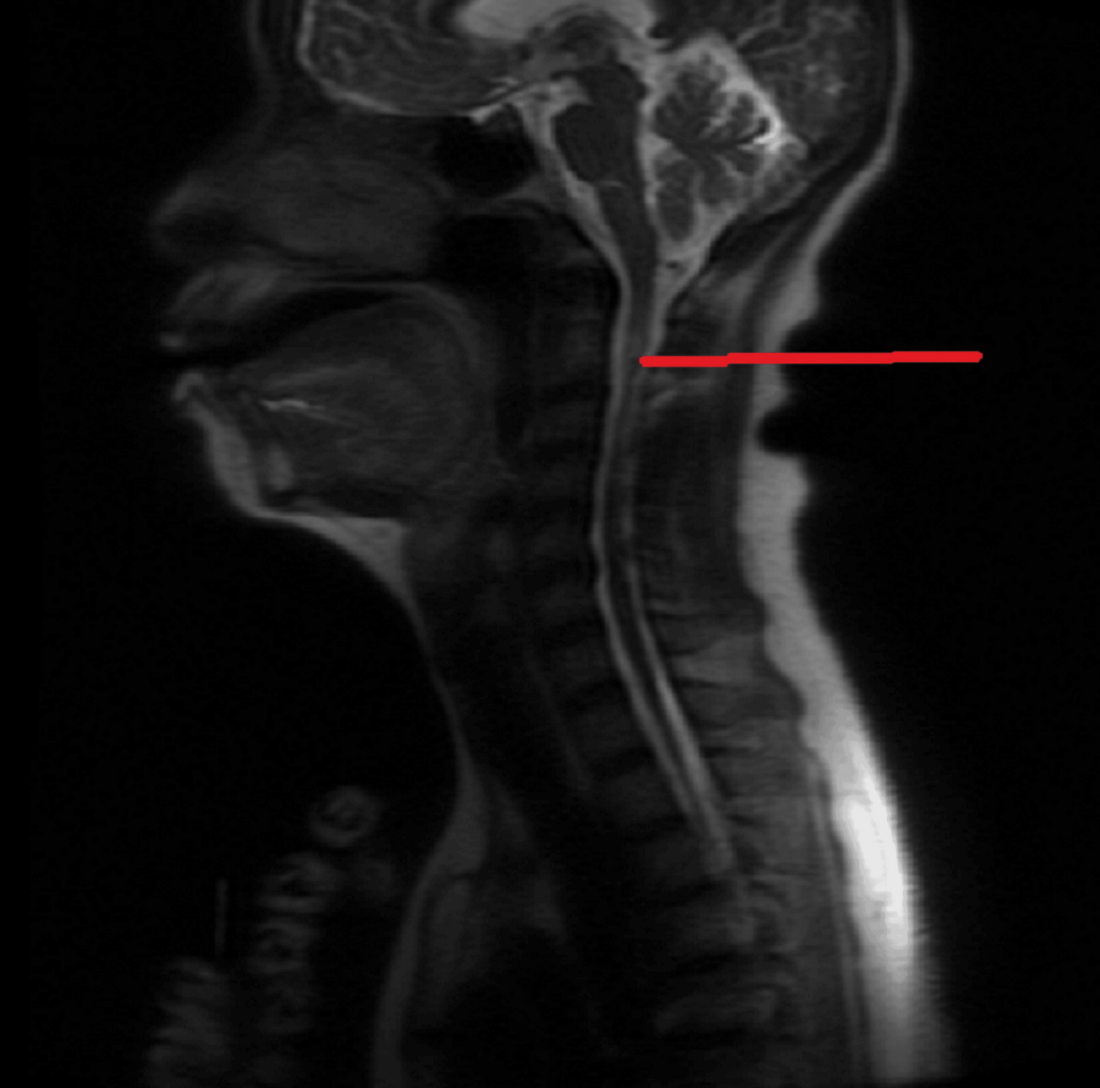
Cervical spine MRI The red line shows lesions within the cervical spinal cord.
